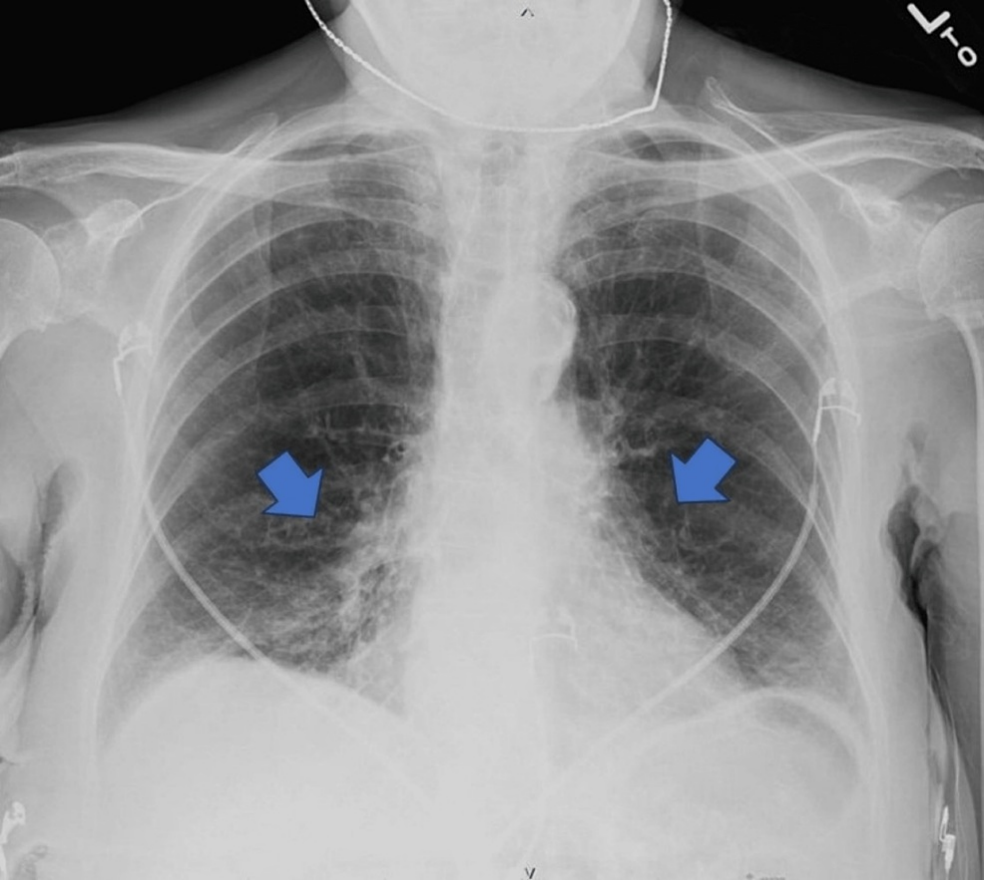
Portable chest radiograph Lungs: well-inflated. Mild chronic interstitial disease with emphysematous changes, stable. Faint mild bibasilar infiltrates. No pneumothorax
